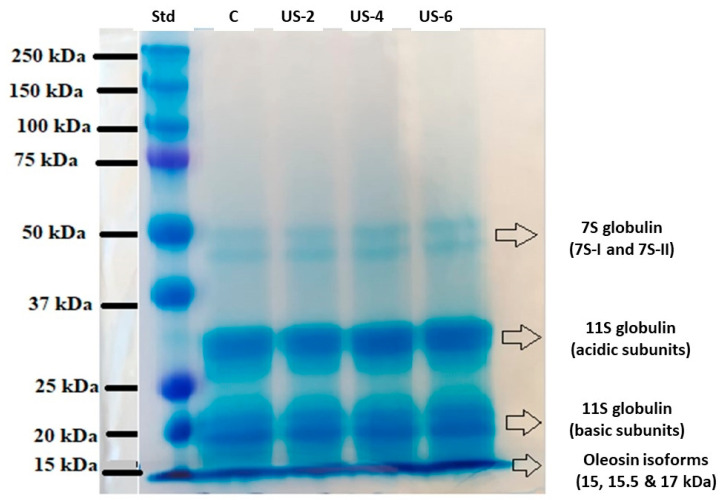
SDS-PAGE profiles of untreated and high-intensity-ultrasound-treated sesame protein isolates. (C, untreated (control); US−2, US−4, and US−6, high-intensity ultrasound treatment for 2, 4, and 6 min, respectively).
